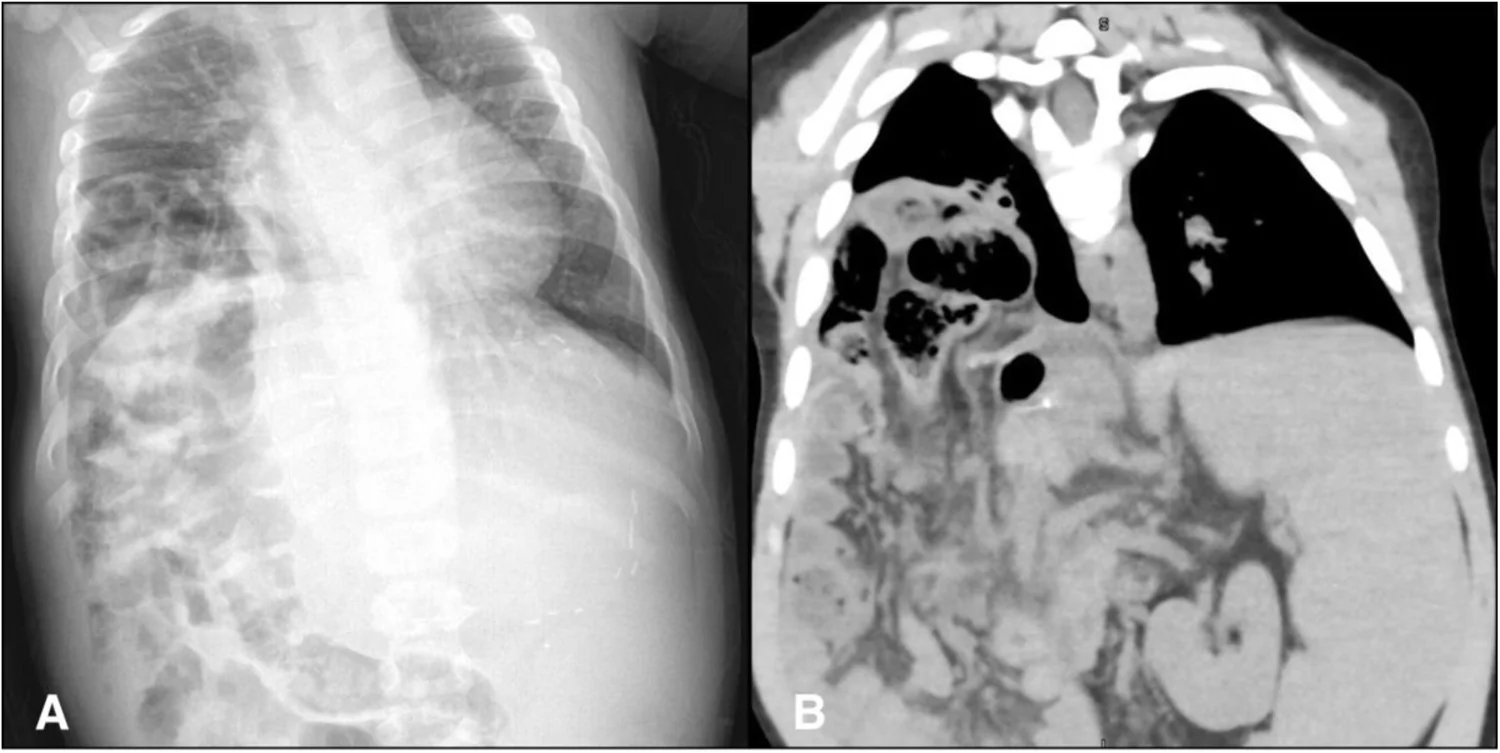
(A) chest radiograph demonstrating bowel gas within the right hemithorax. (B) Coronal CT image confirming herniation of abdominal contents through a diaphragmatic defect.
